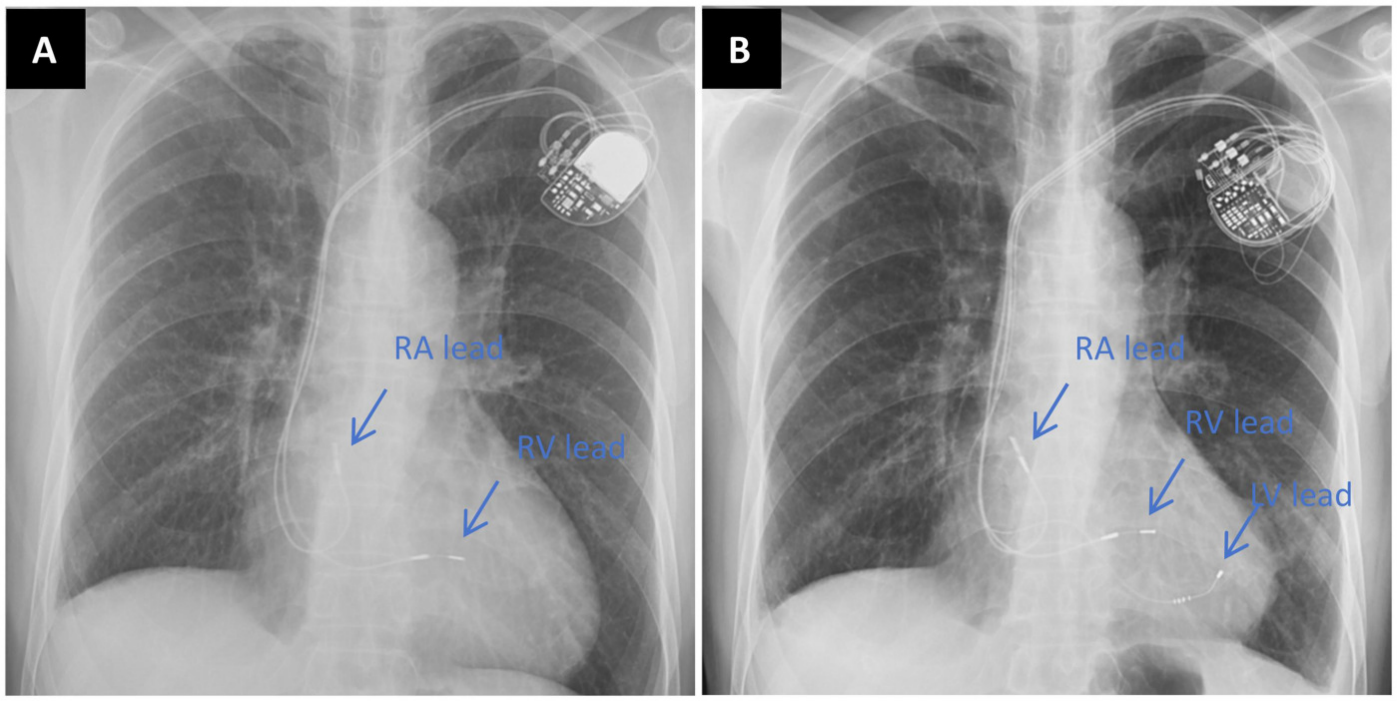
(A) chest radiograph before CRT upgrade. (B) Chest radiograph 1 year after CRT upgrade.
